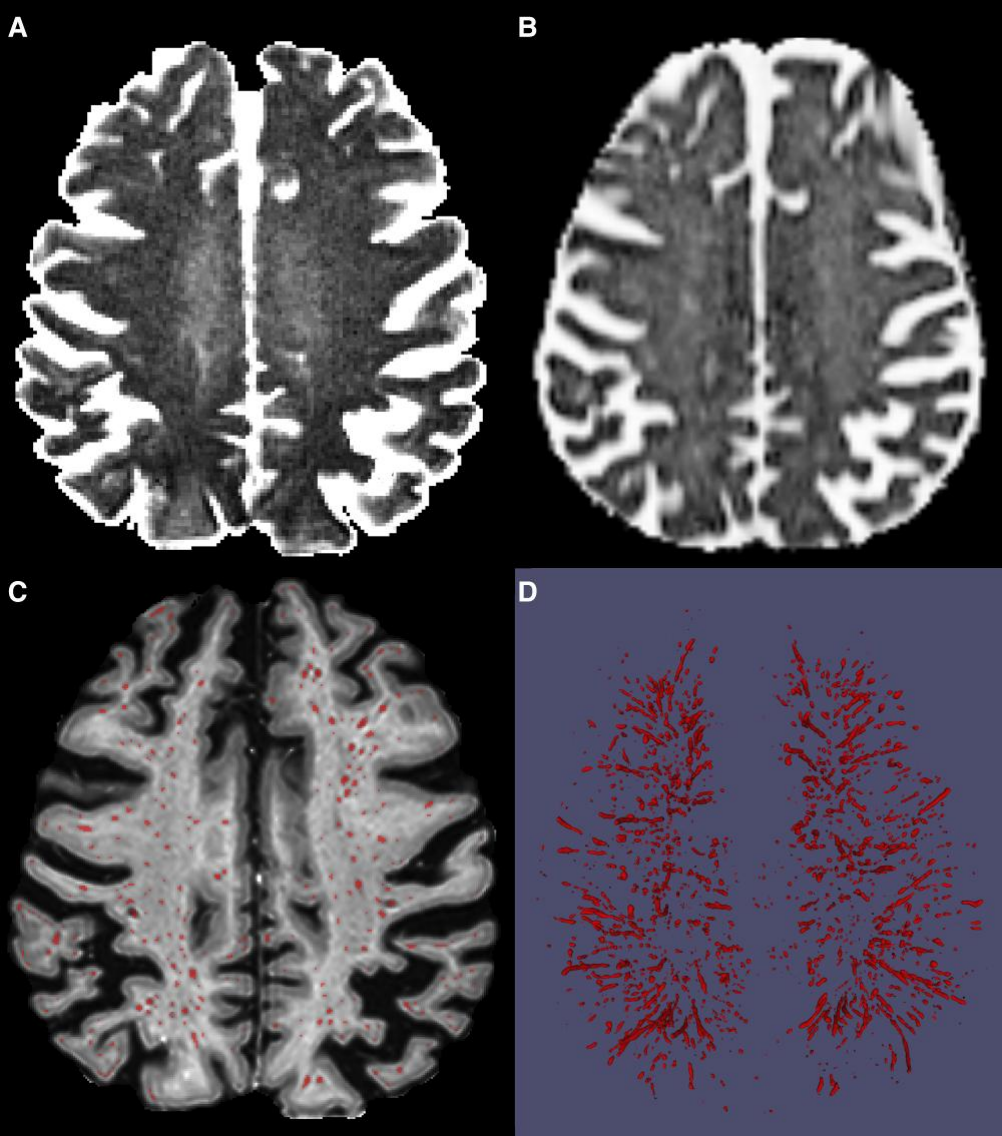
Three types of potential glymphatic fluid volume measures. (A) pCSF corresponding to long T2 signal in three water model fitting based on T2 relaxometry, range [0, 20%], i.e. light region represents higher pCSF value while dark regions indicate lower pCSF; (B) FW corresponding to isotropic diffusion water in bi-tensor DTI model, range [0, 100%] from dark to light; (C) binary PVS segmentation (red) overlayed on EPC map; (D) rendered whole brain 3D PVS volume. Of note the pCSF and FW are whole brain maps, but PVS segmentation is only feasible in WM on 3T MRI and its accuracy depends on image resolution and quality. pCSF, parenchymal CSF; DTI, diffusion tensor imaging; FW, DTI based free-water mapping; PVS, perivascular space; EPC, enhanced perivascular contrast.
